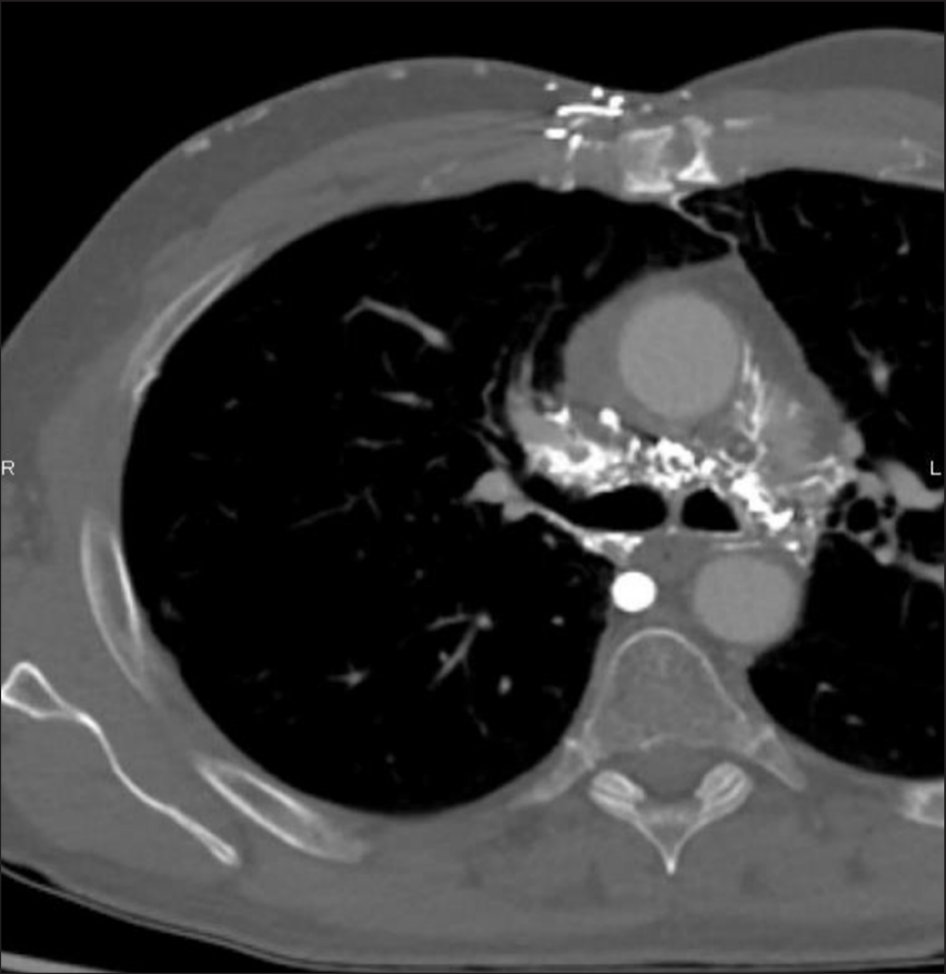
Multiple high-density bone lesions were also detected in several cervical and thoracic vertebral bodies (Figure 3). These findings were not present on a previous CT scan performed two months earlier. Initially these lesions were suggested to be compatible with rapidly progressive bone metastases. A FDG PET-CT showed that these lesions were hypermetabolic (Figure 4).
